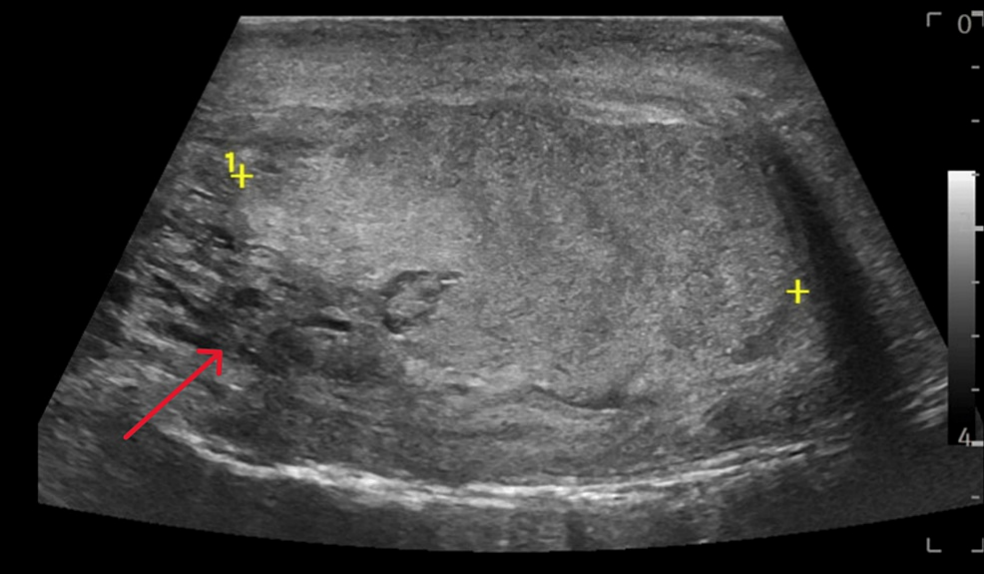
Sagittal grayscale ultrasound of the left testicle approximately 18 hours after injury. Examination shows heterogenous echotexture (red arrow) indicating possible rupture, though continuity of the tunica albuginea appears intact on this series. A diagnosis of testicular rupture was not able to be made based on the findings from this exam.
